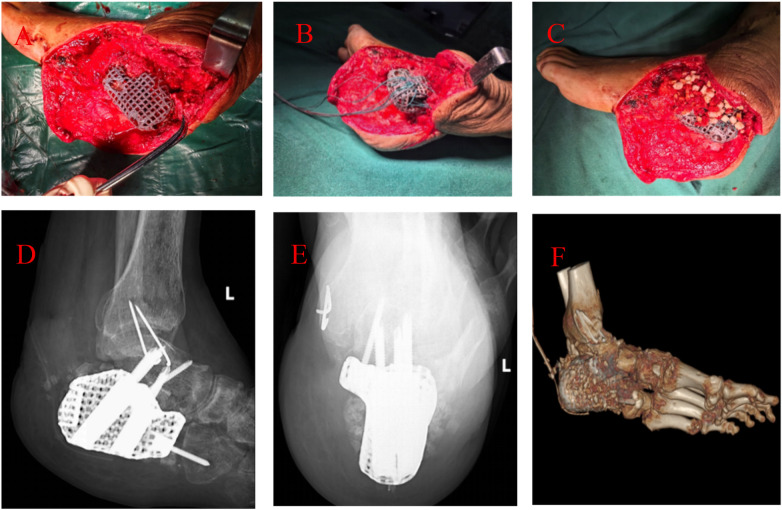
Surgical reconstruction of the calcaneal defect. (A) Implantation of the titanium cage. (B) Intraoperative fixation of the titanium cage. (C) Placement of bone graft material within and around the titanium cage. (D–F) Postoperative radiographic and CT images demonstrating the reconstruction outcome.
